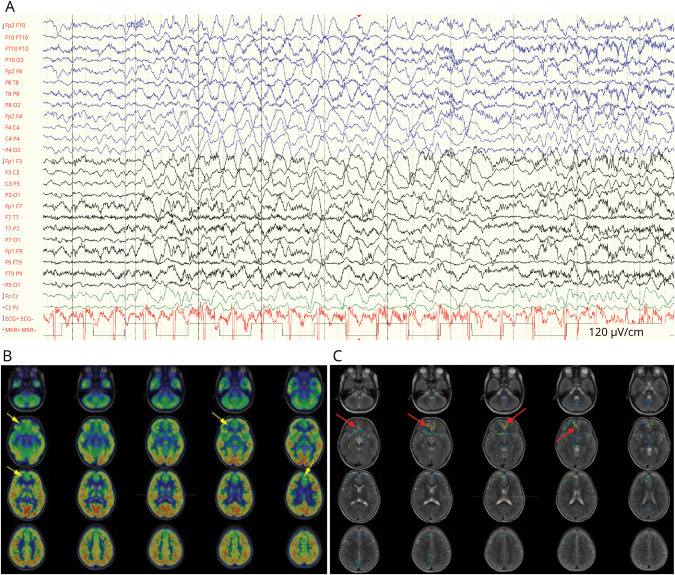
EEG and PET/CT Images in a Girl With Refractory Epilepsy Related to Right Anterior Cingulate FCD Ictal EEG (A) showing increased right frontotemporal activity (gain 120 μV/s with filters 4.0–200.0 Hz). Axial interictal fluorodeoxyglucose (FDG) PET-CT (B) and PET-CT/MRI fusion reconstruction (C) also consistent with an epileptic focus in the right frontomesial region (arrows).
